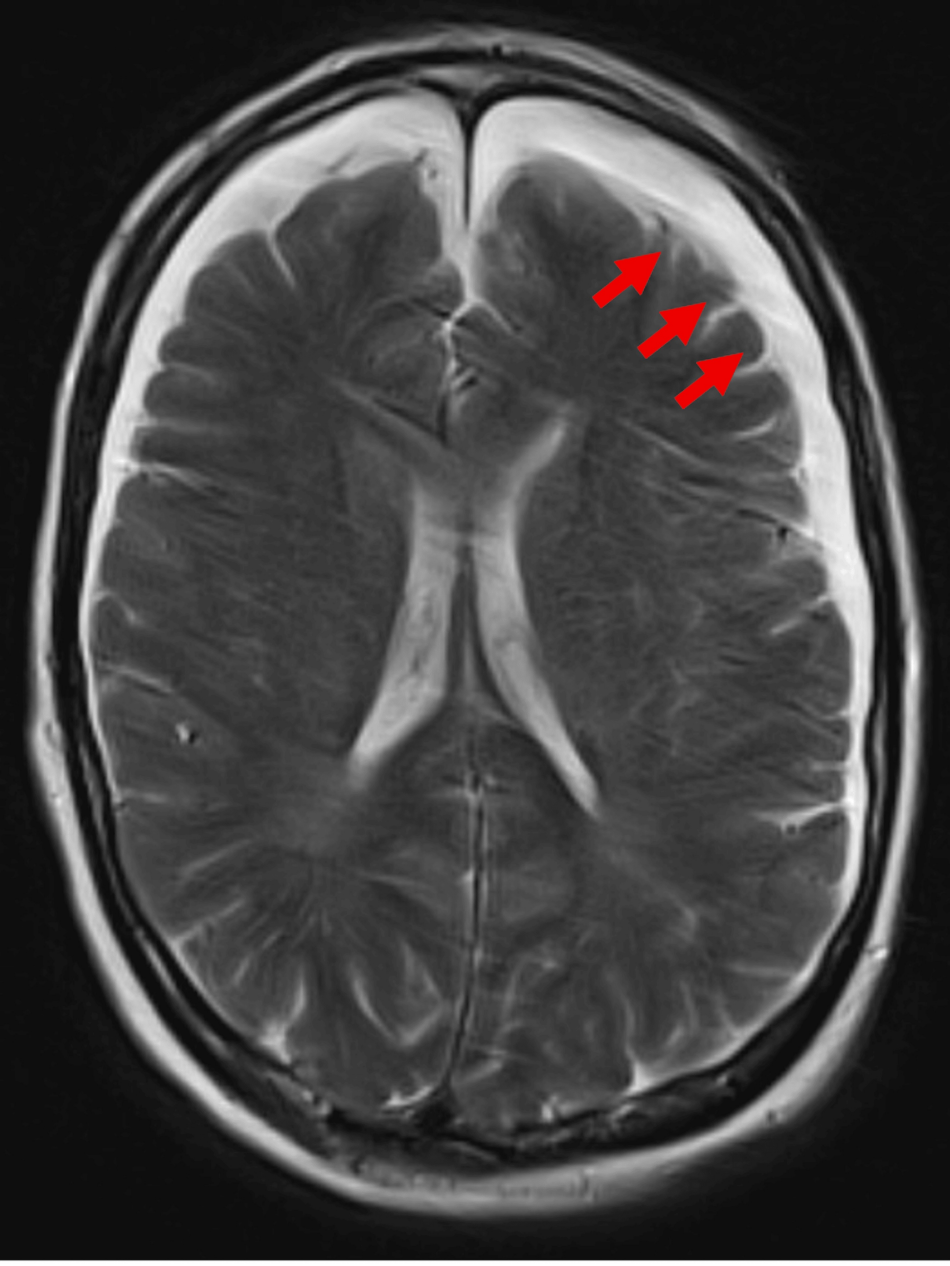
Axial T2 brain MRI showing an acute-on-chronic left frontal subdural hematoma. MRI: magnetic resonance imaging
